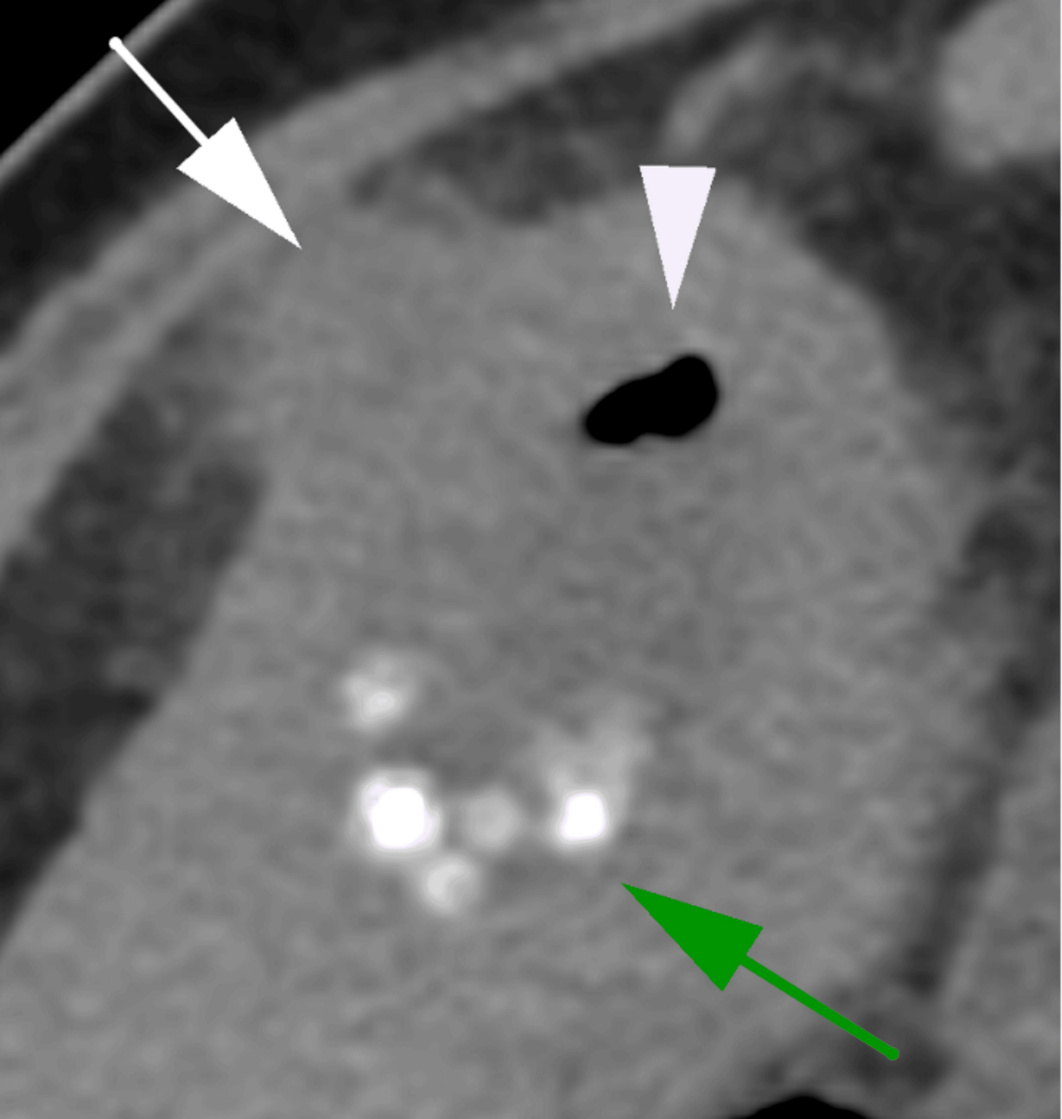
Axial non-contrast CT image of the gallbladder demonstrating gas within the gallbladder lumen (white arrowhead) with associated cholelithiasis (green arrow) and pericholecystic fat stranding (white arrow).
